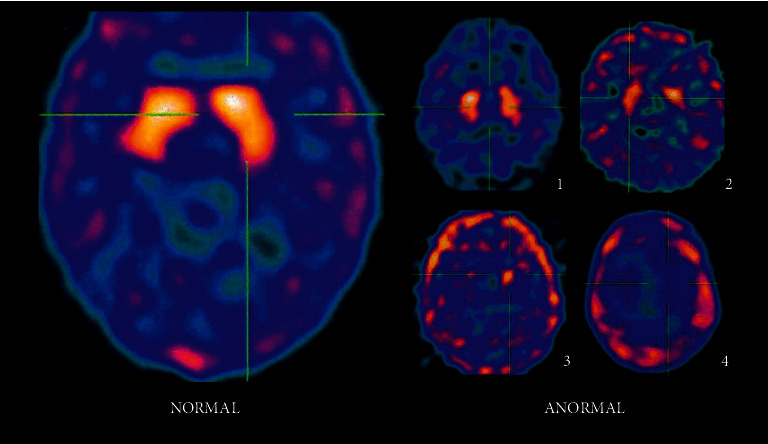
Degrees of involvement according to radiopharmaceutical uptake in cerebral SPECT with ^99m^Tc-TRODAT-1.
